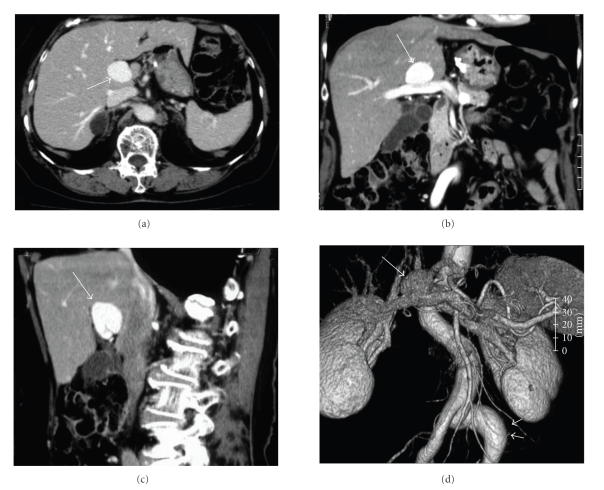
3D-CT (a) horizontal view, (b) coronary view, and (c): sagittal view and CT angiography (d) demonstrated that the saccular aneurysm at the hepatic hilum was 30 mm in diameter and was enhanced equal to that of the portal vein (white arrow). CT angiography demonstrated left common iliac artery aneurysm (white arrow head).
